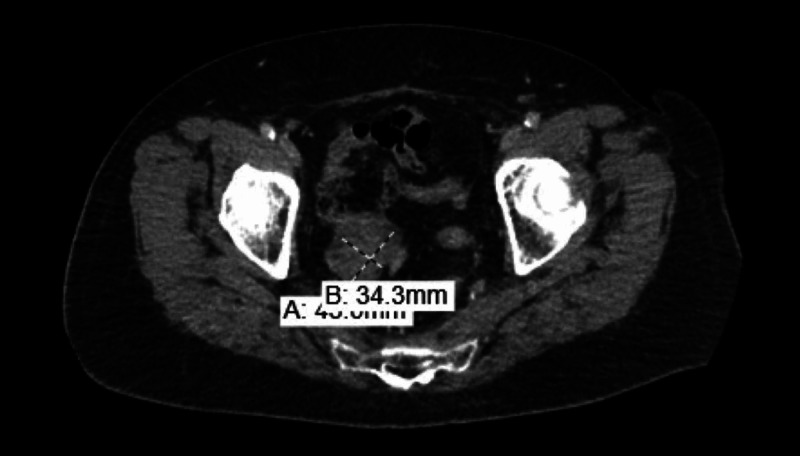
Sagittal view of CT abdomen and pelvis reveals a large solid/cystic pelvic mass surrounding the right ureter
